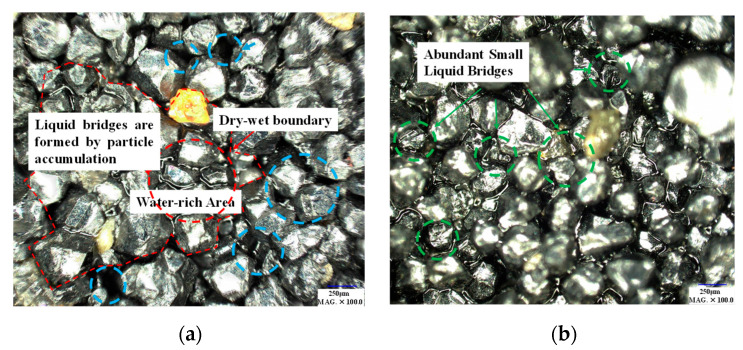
Microstructural distribution of 4.5 wt.% moisture chromite sand preforms at 100× magnification: (a) conventional preform preparation; (b) ultrasonic-assisted preform preparation.
